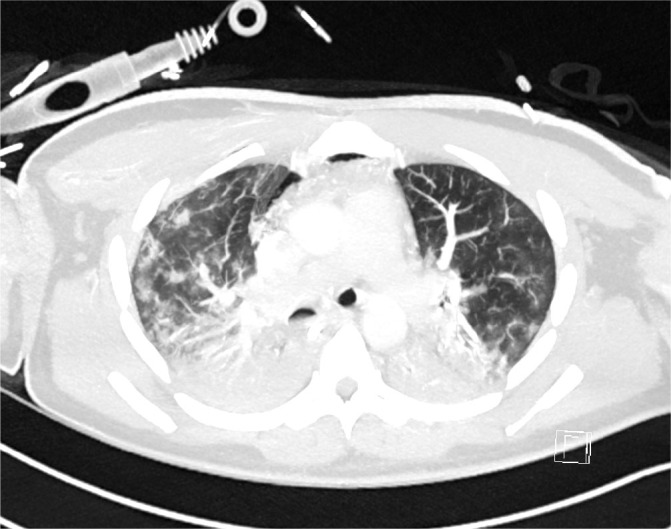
CT Thorax on Day 2 of admission showing extensive bilateral consolidation and surrounding nodular ground glass opacification, in keeping with multi-lobar pneumonia
